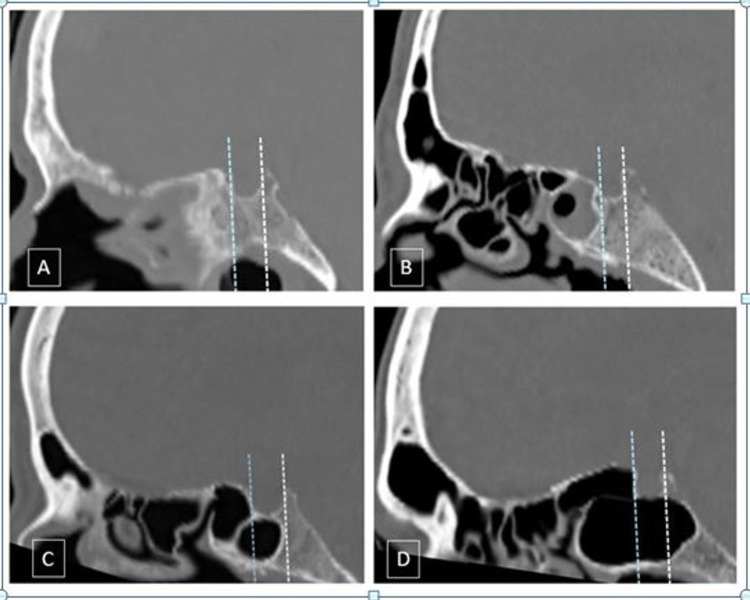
Classification of the posterior extent of sellar pneumatization in the sphenoid sinus CT bone window midsagittal sections showing the various types of sphenoid pneumatization. A: Conchal type; pneumatization (>10 mm) anterior to the anterior wall of sella. B: Presellar type; posterior margin of pneumatization anterior to the anterior wall of the sella. C: Incomplete sellar; posterior margin of pneumatization posterior to the anterior wall but anterior to the posterior wall of the sella. D: Complete sellar; pneumatization extends posteriorly to the posterior wall of the sella CT: computed tomography
